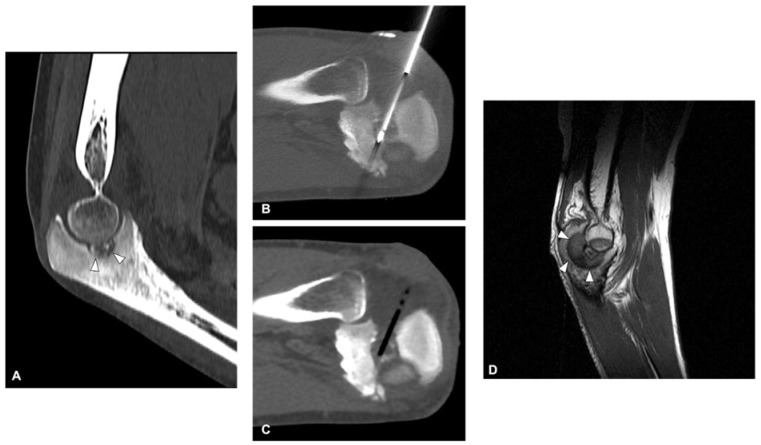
Cryoablation of osteoid osteoma of the elbow in a 28-year-old man: Coronal CT image shows the nidus (arrowheads) with surrounding sclerosis (A). Axial CT images acquired during the procedure show the cryoprobe placed inside the lesion (B) and the low-attenuation ice ball encompassing the lesion (C). Sagittal T1 MRI at 1-month follow-up shows signal change corresponding to the ablation area (arrowheads—(D)). Patient reported considerable improvement in pain.
